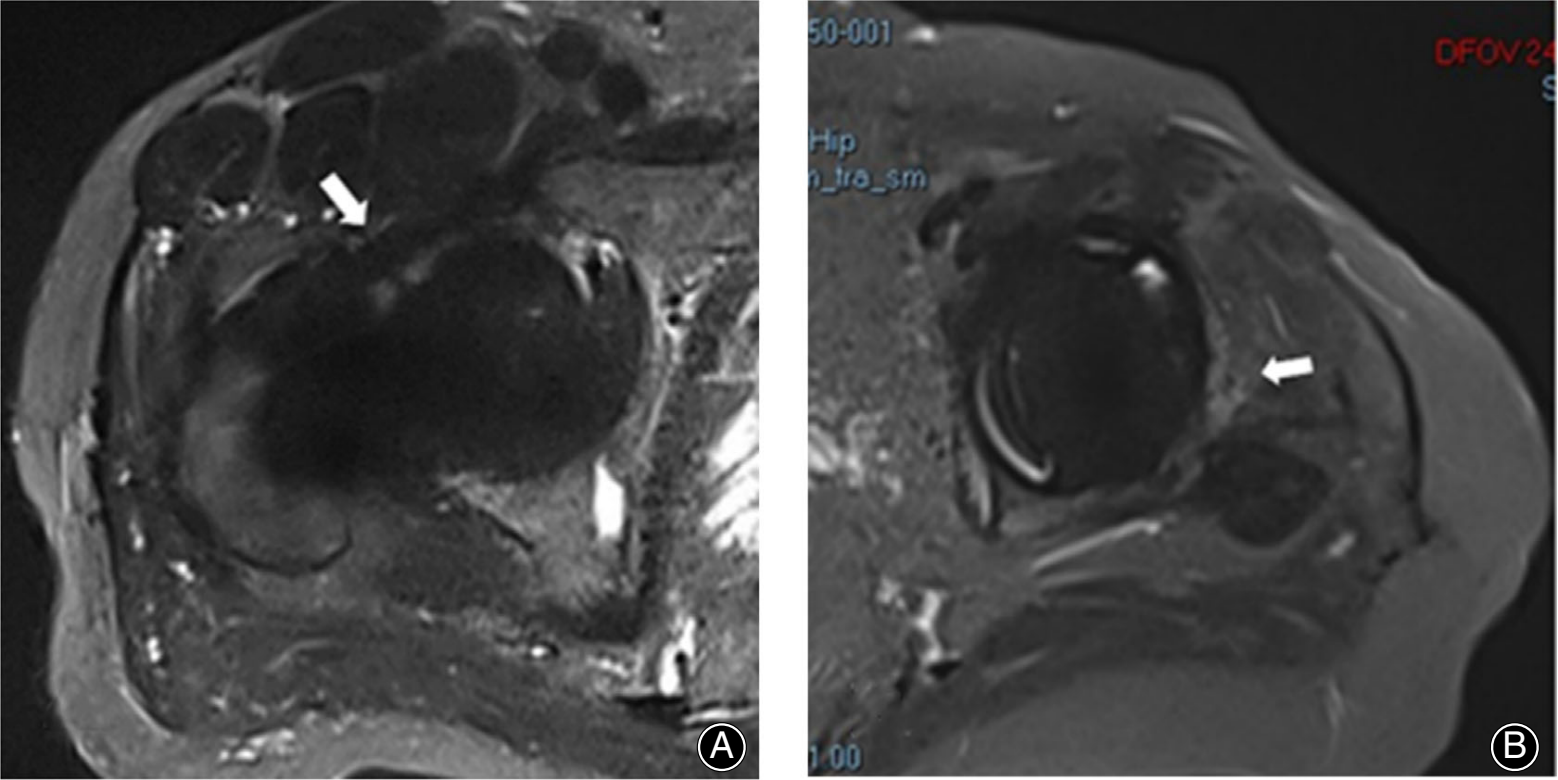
T2WI‐tirm‐SEMAC axial images of two patients underwent THA after MRI examination. (A) Male, 48‐years‐old, 1 year before THA, the postoperative recovery was better without complaints, and the image was obtained at the time of reexamination. A clear articular cavity boundary was observed in front of the joint, and no LHS image was obtained in the axial position of the joint (arrow). (B) Female, 63‐years‐old, 11 years before THA surgery, hip pain for half a year as the main complaint at the time of admission, diagnosis of aseptic loosening of the prosthesis after THA surgery received revision surgery, arrow indicates light high signal soft tissue shadow, but does not have the lamellated characteristics.
